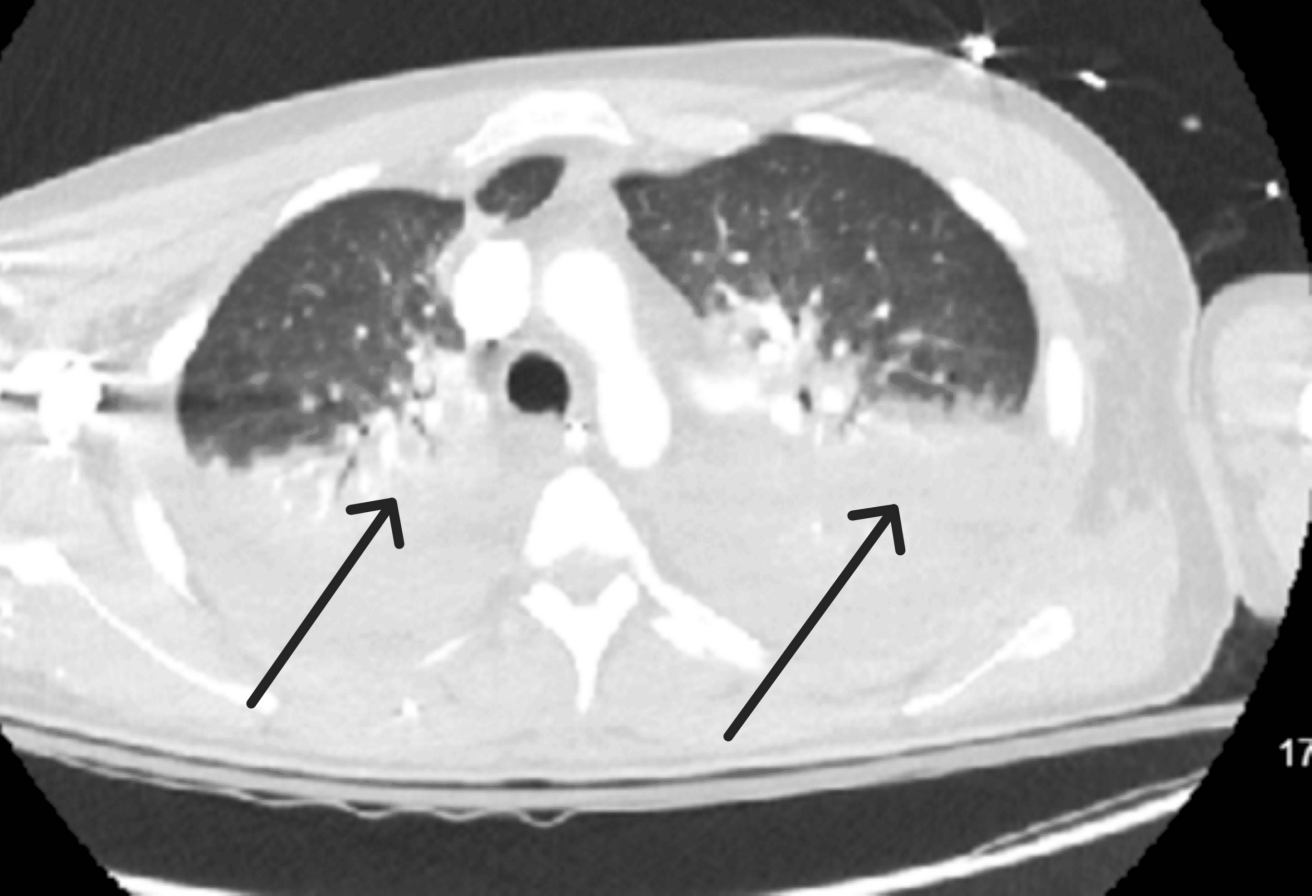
CT chest showing multifocal infiltrates and atelectasis
